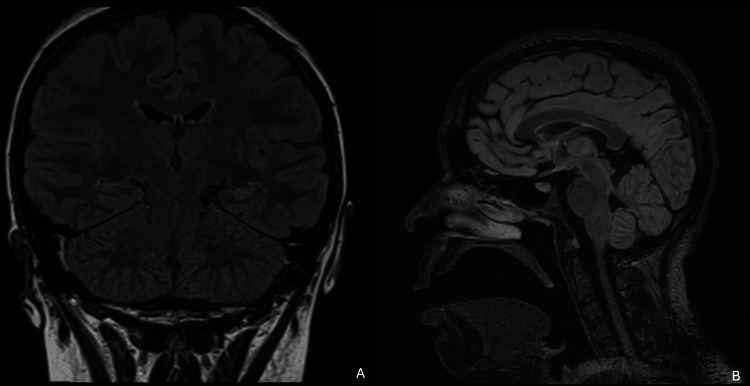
Brain MRI. Brain MRI demonstrating no structural abnormalities. (A) Coronal fluid-attenuated inversion recovery (FLAIR) sequence. (B) Sagittal T2-weighted sequence.
